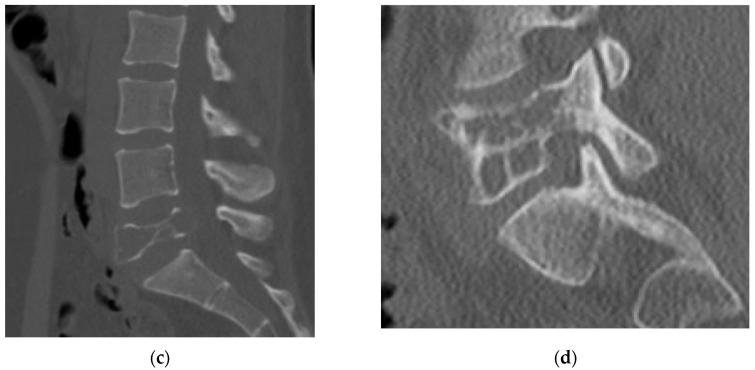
(a) Axial CT cuts of L5 vertebral body shows pseudotrabeculation of vertebral body with eccentric thinning of the right vertebral body cortex and formation of a contralateral sclerotic margin. (b) Bilateral pedicle involvement with near complete destruction of right sided L5 pedicle. (c) Sagittal cuts of L5 vertebral body shows loss of vertebral height indicating compression fracture with no retropulsion of bony elements. The lesion is seen to by lytic with cortical erosion especially in the posterior vertebral body. (d) Sagittal cut of L5 vertebral body demonstrates classic “soap bubble” imaging characteristic seen on the vertebral body of L5 on CT scan.
